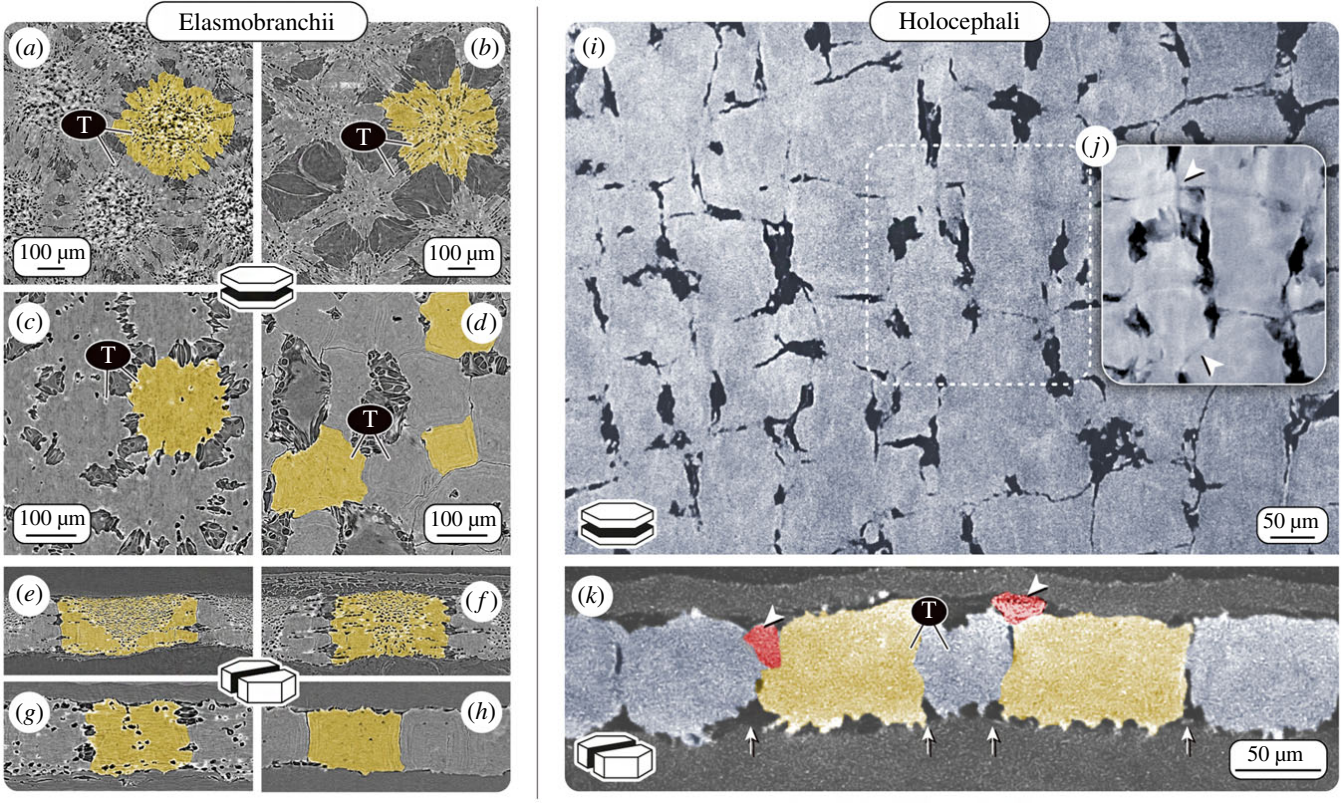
Variation in tessellation patterns in elasmobranchs and holocephalans. Computed tomography virtual sections of tesserae (T; with individual tesserae coloured yellow) from four different elasmobranch species: (*a,e*) a skate, *Raja stellulata*; (*b,f*) a stingray, *Pteroplatytrygon violacea* and two sharks (*c,g*) *Heterodontus francisci*; (*d,h*) *Notorynchus cepedianus*; and a holocephalan species (*i–k*) *C. monstrosa.* Images *a–d* and *i,j* show virtual planar sections, *e–h,k* are virtual vertical sections of tesserae. (*i*) The tiling pattern of skeletal mineralization in the chondrocranium of *C. monstrosa* appears most similar to that of the broadnose sevengill shark *Notorynchus cepedianus* (*d,h*), with tesserae exhibiting irregular geometric shapes and almost no cellular lacunae (visible as black dots in most of the elasmobranch tesserae). The presence of cell lacunae is considered the standard condition for (*a–h*) elasmobranch tesserae. (*j*) Averaged planar section created from six consecutive sections, revealing tesserae borders (intertesseral joints), which were not clearly visible in (*i*) a single section plane. (*k*) In vertical sections, some adjacent tesserae in *C. monstrosa* overlap (red tesserae, marked with arrowheads), whereas others are in contact at their lateral edges (intertesseral joints, marked with arrows). (Images *a–h* adapted from [23] with permission from Wiley.)
